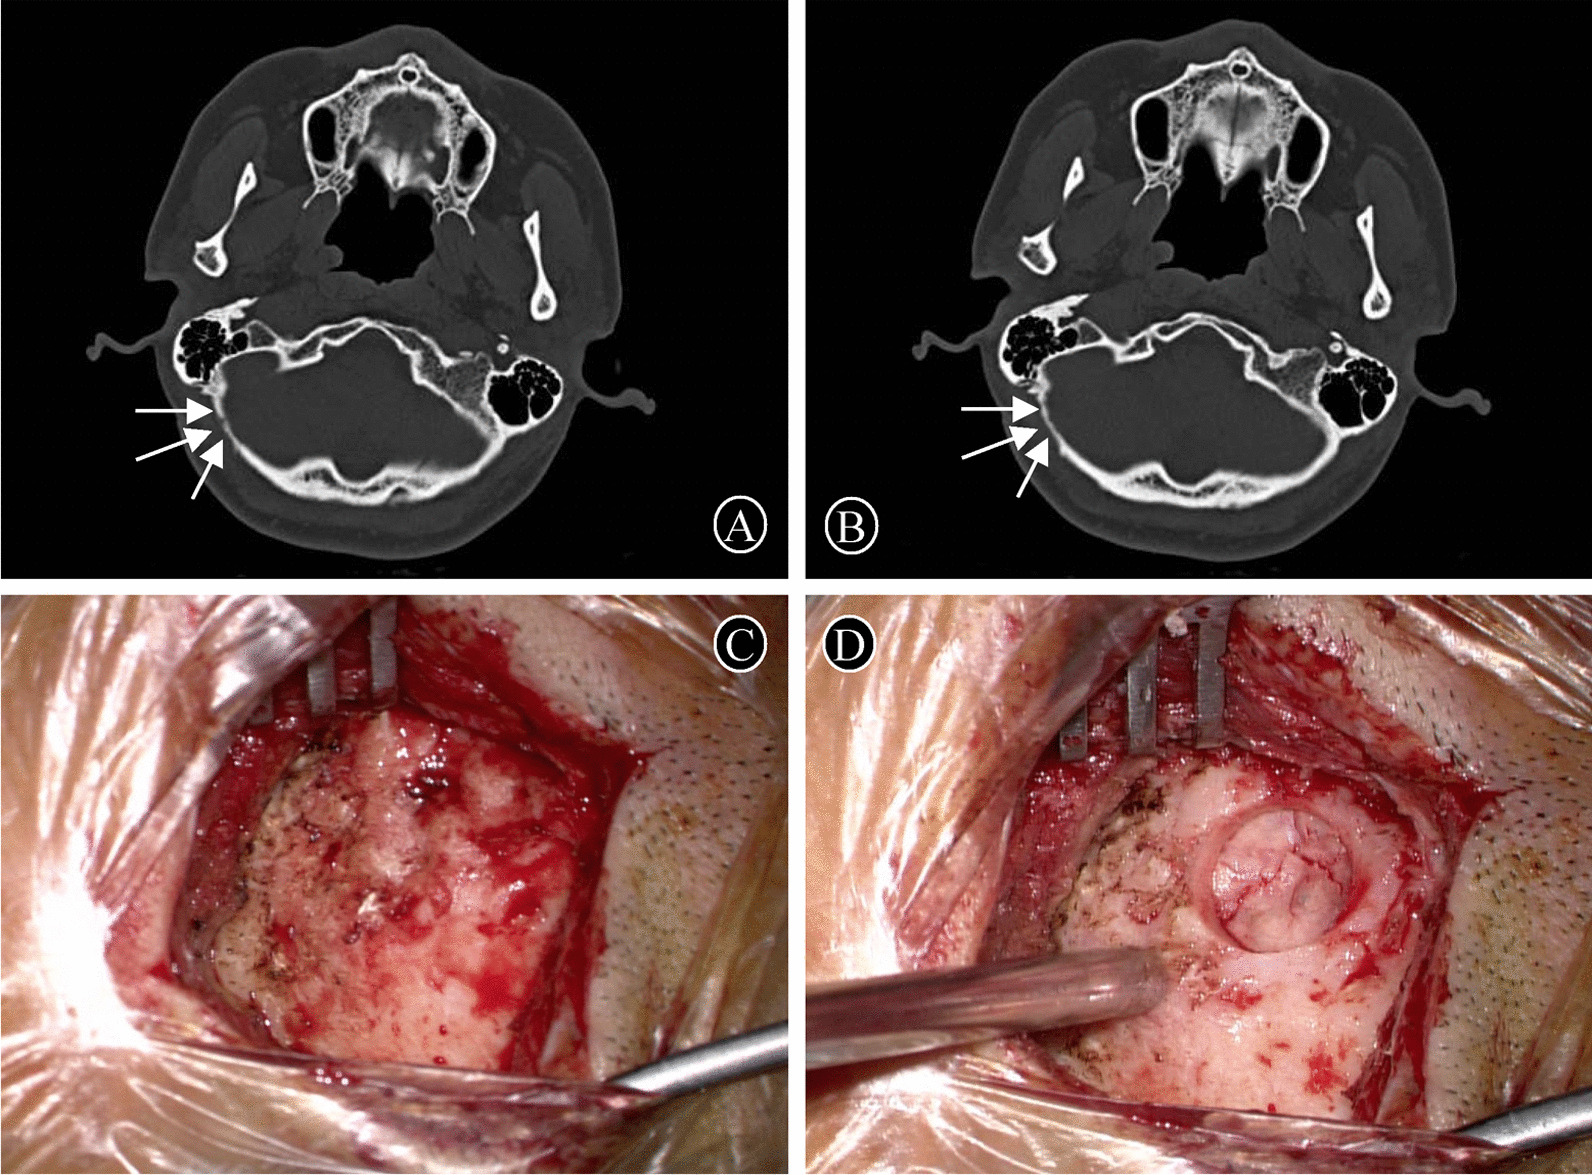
Cranial CT and intraoperative findings at one year after skull reconstruction. A, B The skull defect was completely reconstructed on cranial CT one year after the skull reconstruction (white arrow). C, D A second MVD one year later showed that the new skull bone filled the skull defect caused by the previous operation
